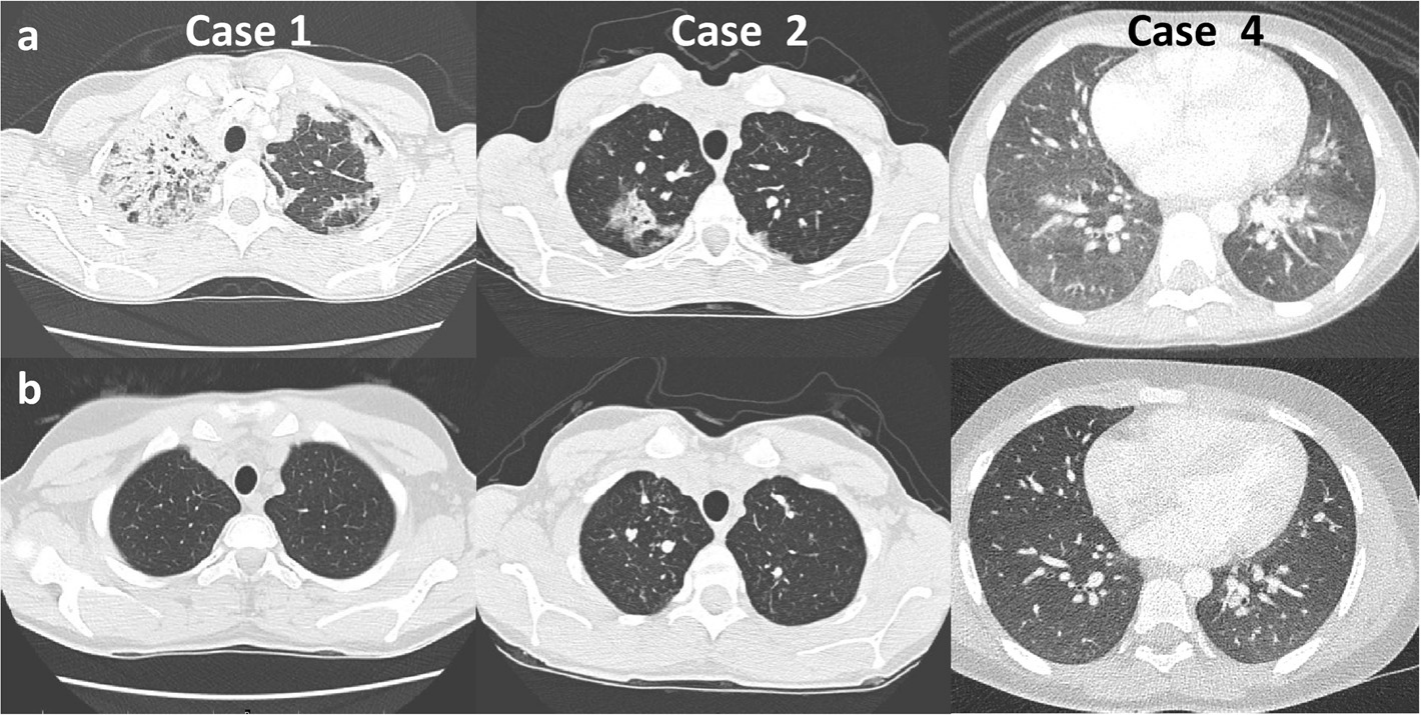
HRCT scan in “dramatic improvement subset” ICEP patients. (a) At diagnosis: in case 1, bilateral and peripheral, dense and fluffy alveolar opacities associated with ground-glass opacities predominantly in the right superior lobe; in case 2, peripheral, alveolar, dense and fluffy opacities with areas of ground-glass opacities predominantly in the right and also in the left apex; in case 4, patchy ground-glass opacities and nodules. (b) Last HRCT scan showing complete normalization.
